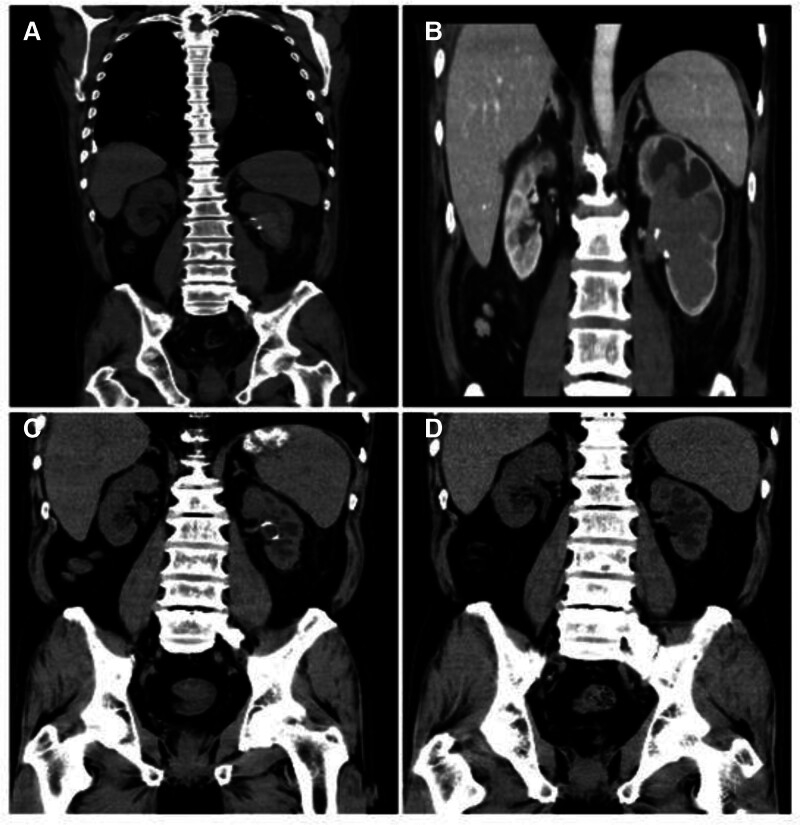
CT changes after the second renal hemorrhage. (A) Significant hematoma in the left renal pelvis after heparinized dialysis at an external hospital. (B) After non-heparinized dialysis, the left kidney showed no arteriovenous fistula or pseudoaneurysm formation on CT enhancement. (C) Hematoma in the left renal pelvis resolved spontaneously after dialysis was stopped. (D) Four months post-surgery, removal of the double-J stent showed no significant worsening of left renal hydronephrosis. CT = computed tomography.
